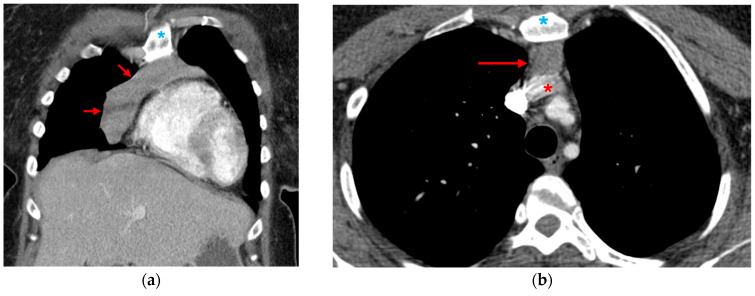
Thymic hyperplasia. (a,b) Axial CTA images show a mediastinal, homogenous soft tissue density (red arrow) positioned in the anterosuperior mediastinum immediately anterior to the aortic arch (red asterisk) behind the manubrium (blue asterisks). The location, characteristics, and smooth lobulated margins are consistent with thymic hyperplasia.
